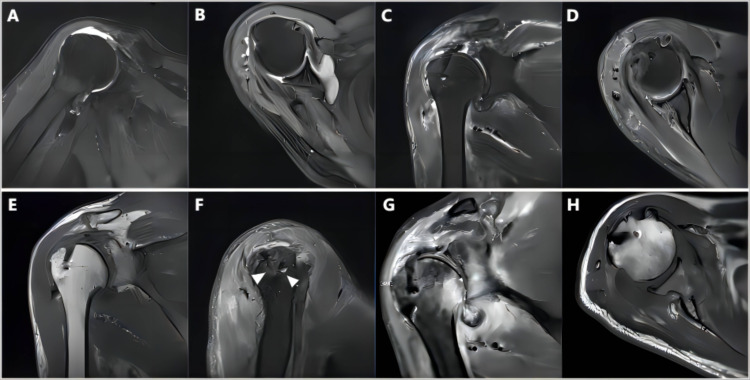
MRI images before and after surgery. A, B: Preoperative MRI images; C, D, E, F: MRI images 3 months post-surgery, where C shows the coronal plane in the T2 phase, D is a cross-sectional MRI, E represents the coronal plane in the T1 phase, and F displays the MRI in the sagittal plane with bone tunnels indicated by arrows; G, H: MRI images 6 months post-surgery.
